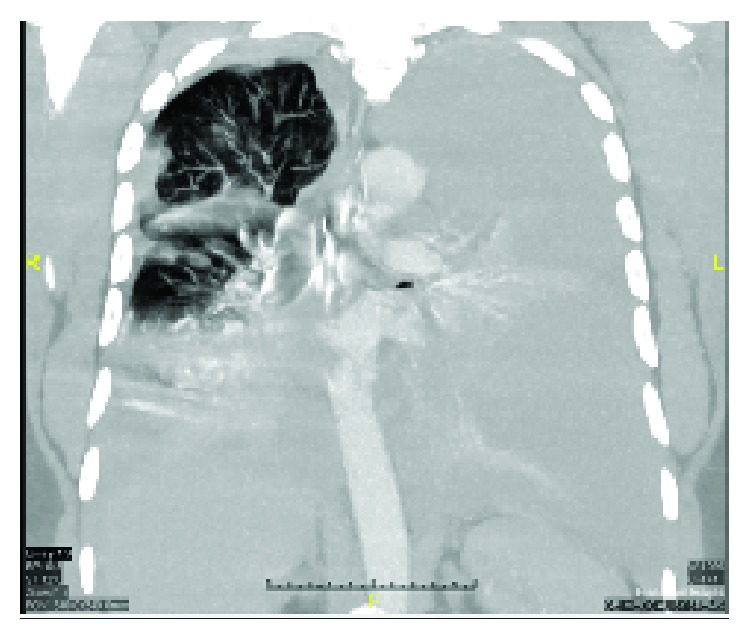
Initial CT chest of the patient (coronal view) with complete obliteration of the left hemithorax and some lung parenchyma seen in the right hemothorax. Hounsfield units were consistent with hemorrhagic effusion.
